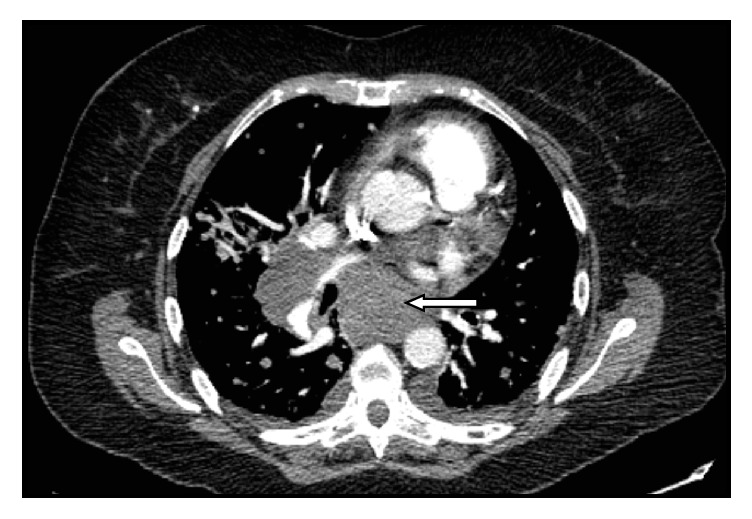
Preoperative computed tomography (CT) scan. This CT demonstrates large mediastinal and hilar lymph nodes with the largest being located in station VII (subcarinal nodes) with a 5.6 cm diameter (arrow) resulting in a mass effect on the bronchus and hilar vessels.
